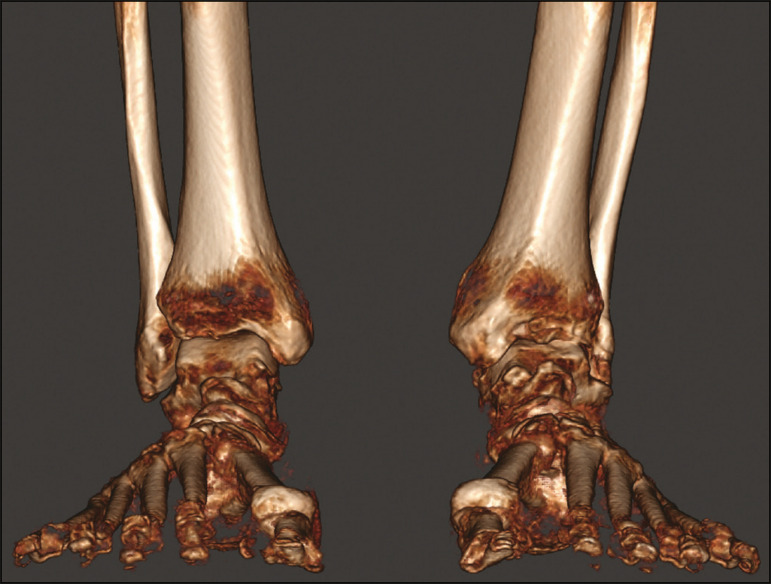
3D reconstruction for bone assessment, achieved with CubeVue software after volumetric acquisition with weight-bearing CBCT.
